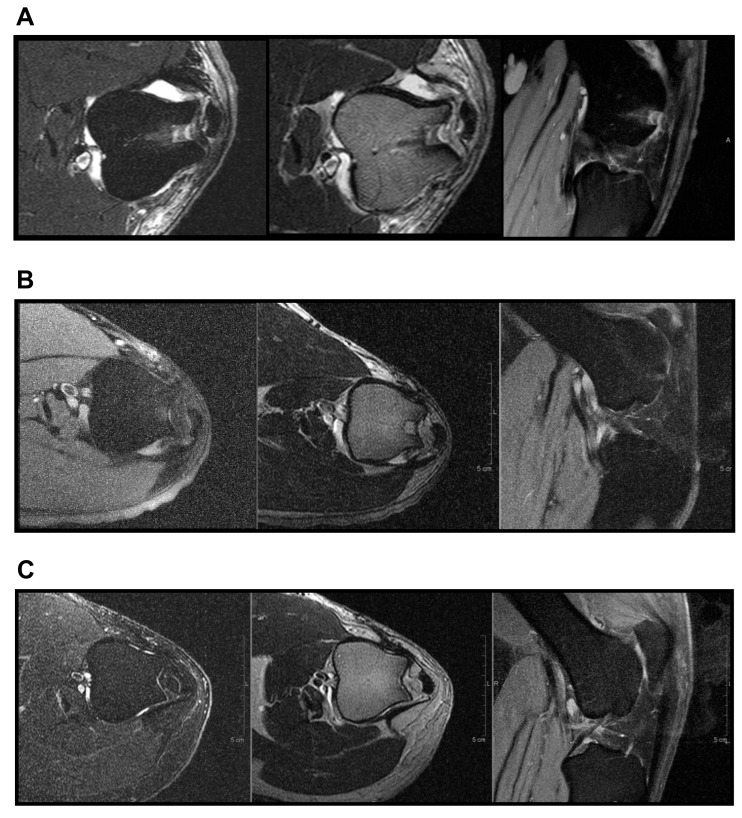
Reference MRI images, from left to right: axial PD fs, axial T1 without fs, sagittal PD fs. (A): MRI after 30 days, “PD Signal Intensity” was rated 3, “T1 bone mineralization” was rated 2. (B): MRI after 180 days, “PD Signal Intensity” was rated 7, “T1 Bone Mineralization” was rated 6. (C): MRI after 365 days, “PD Signal Intensity” was rated 10, “T1 Bone Mineralization” was rated 10.
